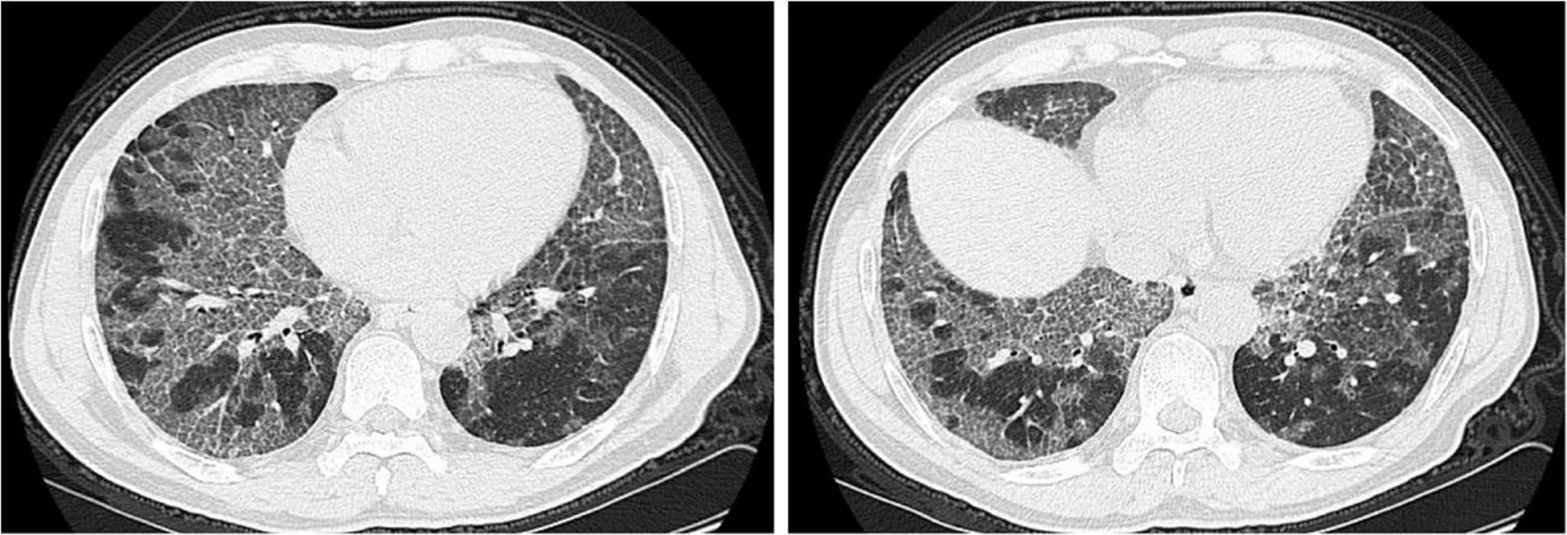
Chest CT image before whole lung lavage showing a bilateral ground-glass opacity with interlobular septal thickening with a typical crazy paving pattern.
